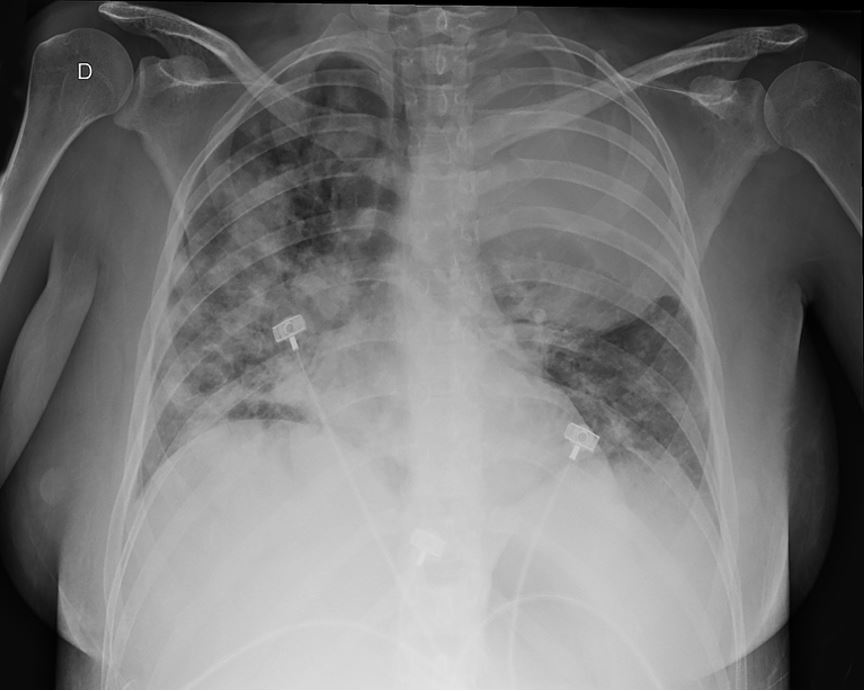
Chest radiography in day 4 of admission, prior to ICU admission, and subsequent orotracheal intubation. D stands for right side.
